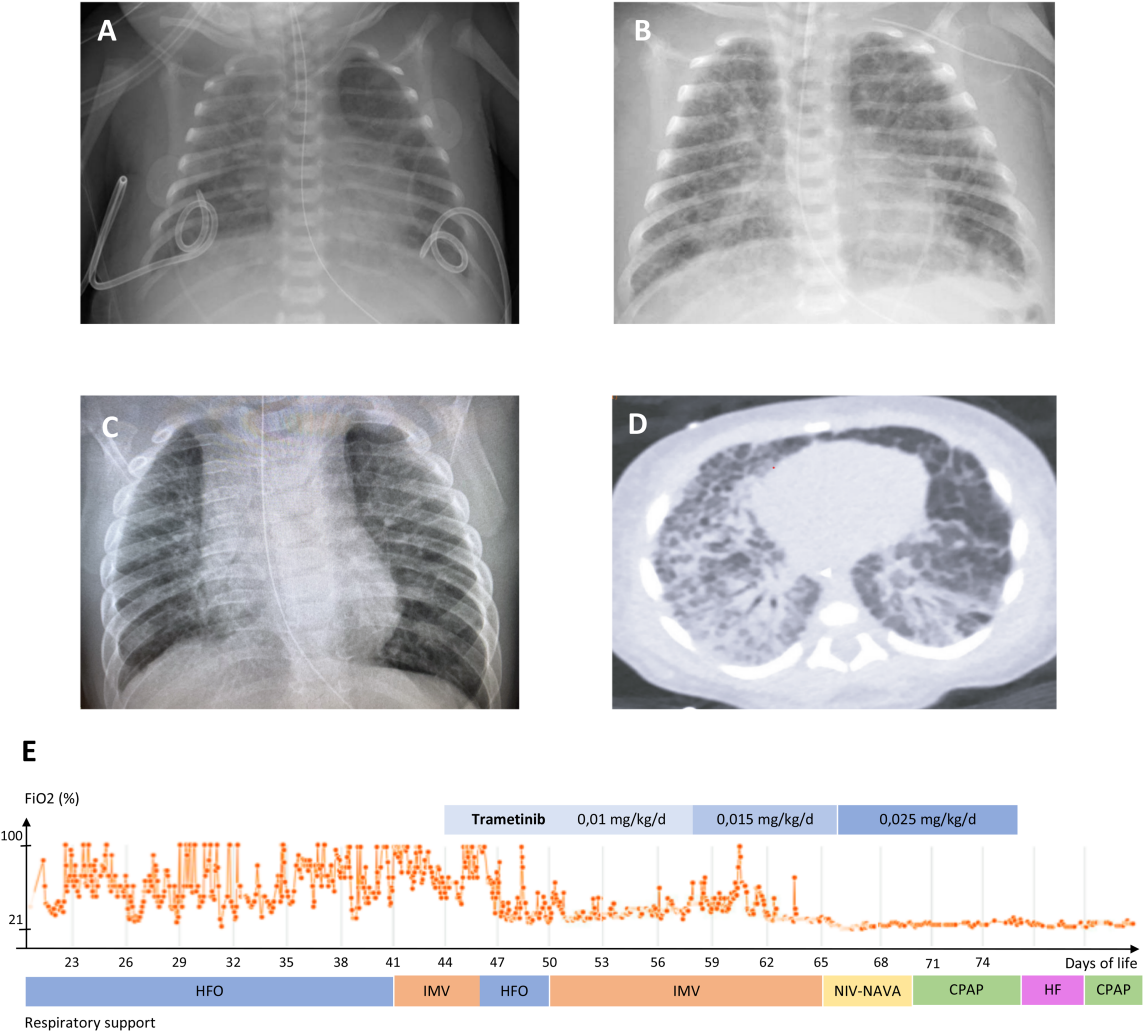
(A–C) chest radiograms of patient #1: (A) 22 days of life; (B) 2 months; (C) 12 months. (D) Chest computed tomography scan, 32 days of life. E: mean daily FiO_2_ during level 4 neonatal intensive care stay, ventilation mode and trametinib regimen. HFO: high frequency oscillatory ventilation; IMV: intermittent mandatory ventilation; NIV-NAVA: non-invasive neurally-adjusted ventilatory assist; CPAP: continuous positive airway pressure; HF: high-flow nasal cannula.
